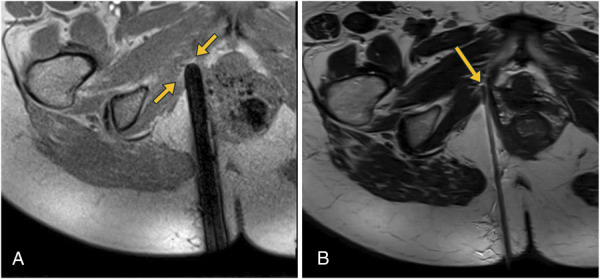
Target confirmation with the trocar adjacent to the anchor. A. High T1-weighted gradient echo signal attributable to enhancement from gadolinium (*yellow arrows*). B. High T2-weighted signal (*yellow arrow*) attributable to the water in the methylene blue dye/lidocaine/gadoteridol solution. Note the exaggerated needle artifact on T1-weighted imaging, which is why T2-weighted imaging was used for most of the needle advancement.
